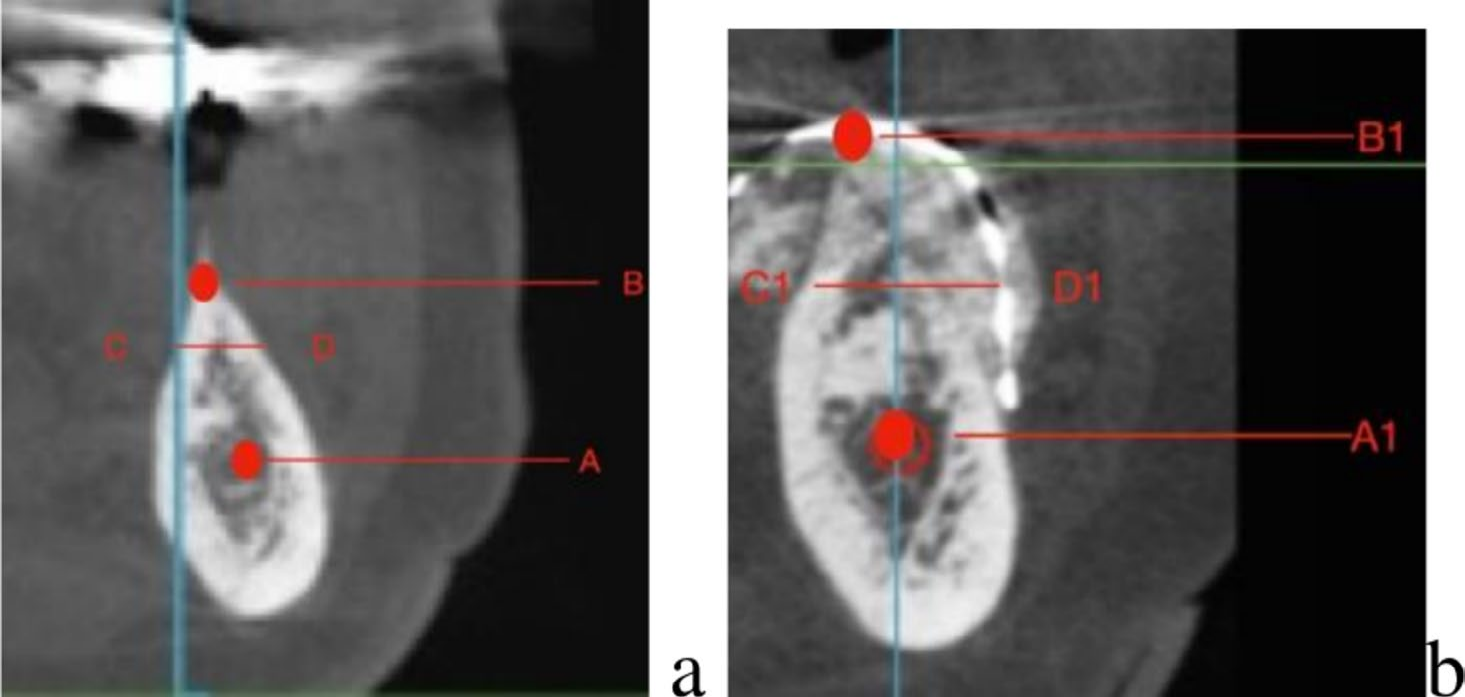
(a) Preoperative CBCT sagittal cut. (b) postoperative CBCT sagittal cut. Landmarks A and A1 identify the position of the alveolar canal, while B and B1 the most coronal point of the crestal height. The distance A → B and A1 → B1 are the preoperative and postoperative heights of the defect. Similarly landmarks C and C1 identify the lingual point used for the measurement of the mandibular or maxillary width, while D and D1 the buccal point. Therefore the distance C → D and C1 → D1 are the preoperative and postoperative widths of the defects.
